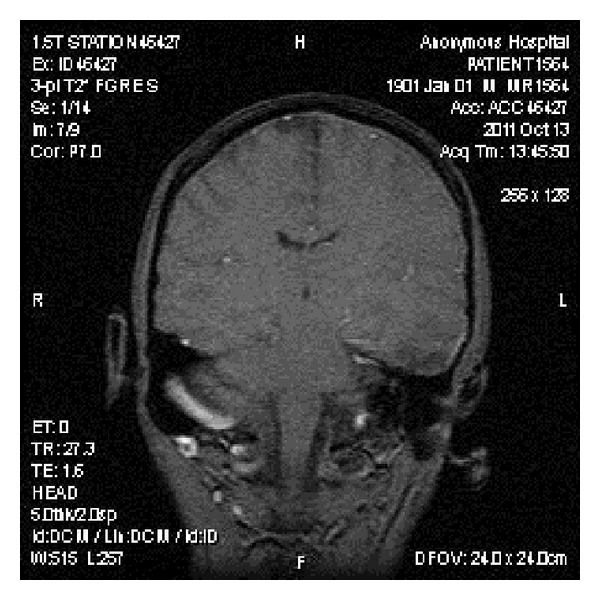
Brain MRI with and without contrast showing bihemispheric subdural hematomas.
